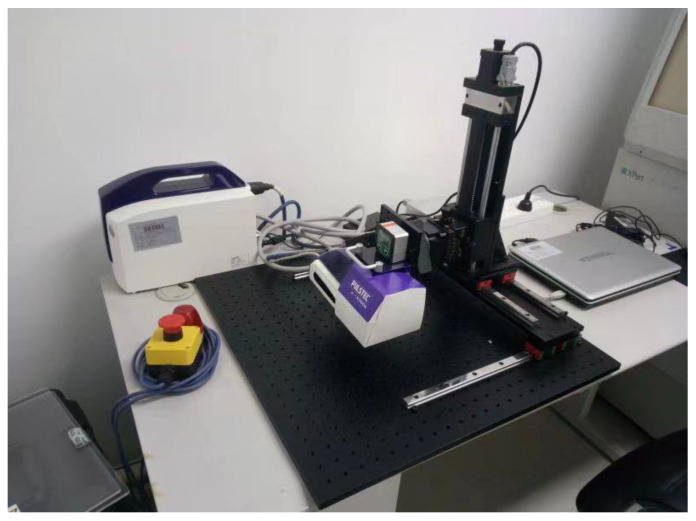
Pulstec μ-X360s X-ray residual stress analyzer.
